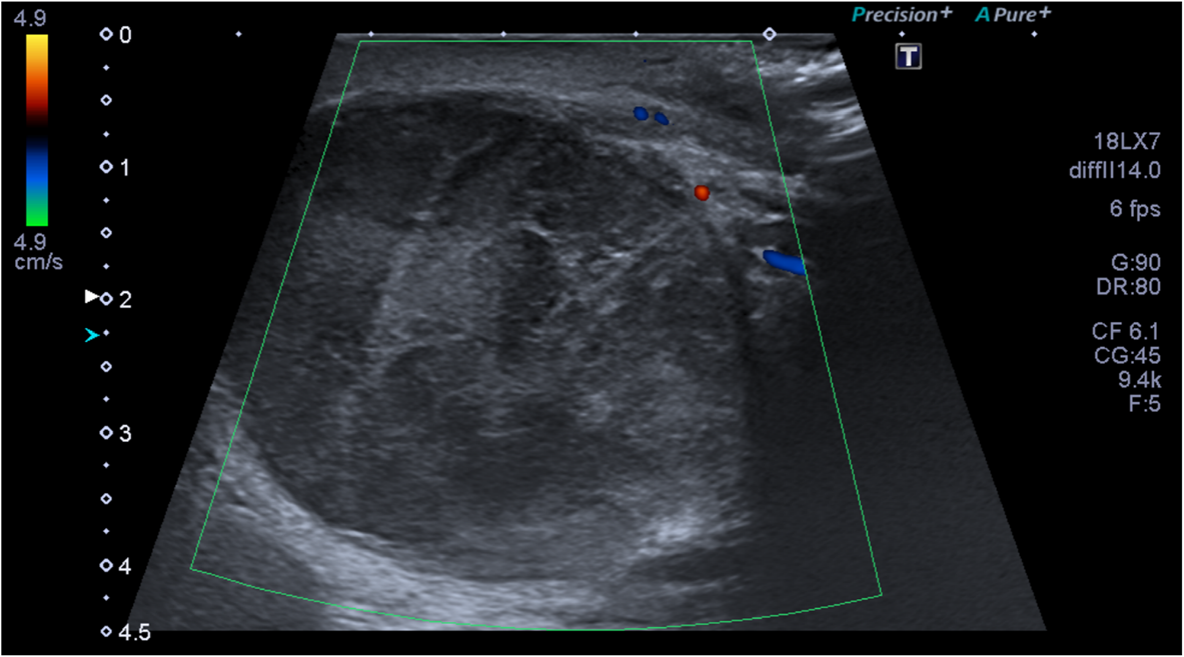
Colour Doppler Ultrasound of the same testis left, 2 months after presentation. There is progression of the size of the mass to 40×40mm. The swelling is not demarcatable from the left testis. Although there is no vascular flow visible, due to the progression in size and the aspect of the swelling, a malignancy cannot be ruled out
